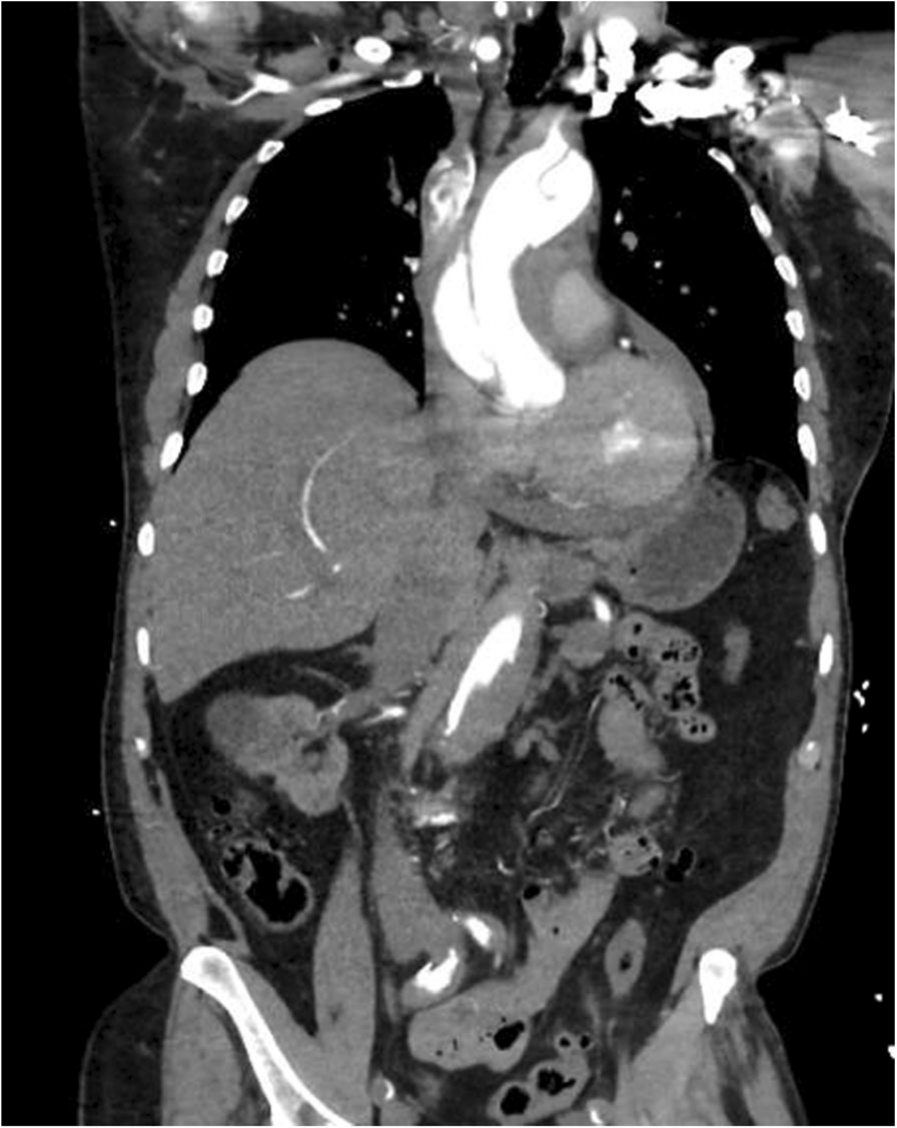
CT angiography of the chest, abdomen, and pelvis with and without intravenous contrast revealing a Stanford type A dissection with an intimal flap seen beginning at the level of the aortic root and extending throughout the course of the thoracic aorta and extending into the abdominal aorta
